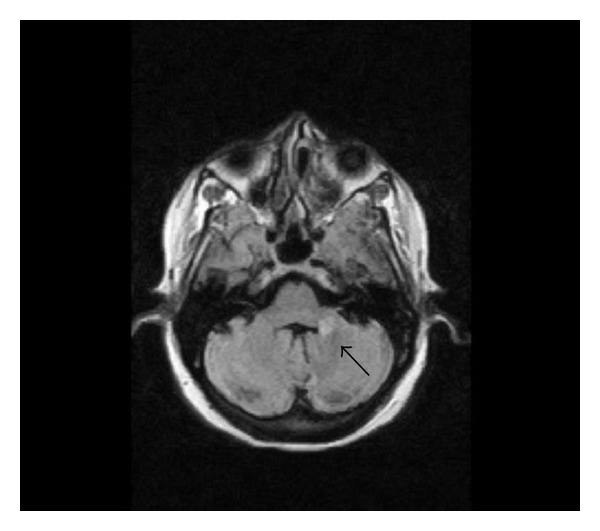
Axial T2 FLAIR image shows small hyperintense lesion in the left middle cerebellar peduncle.
